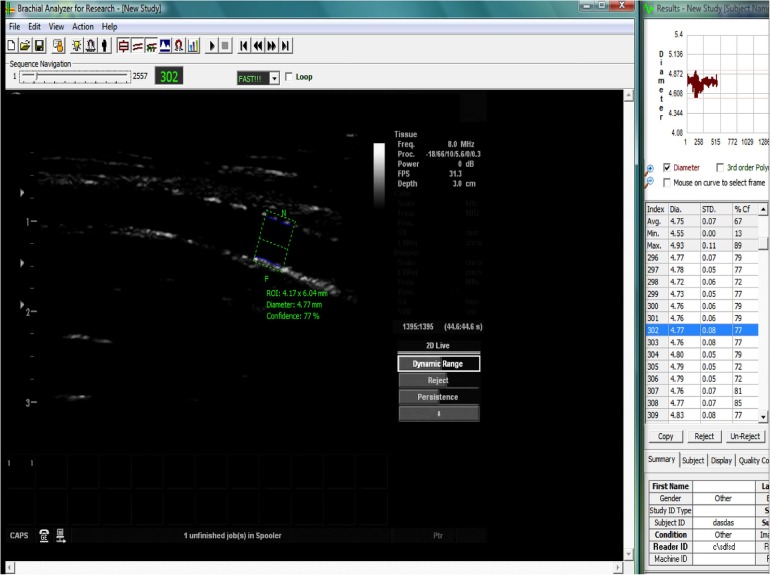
Screen shot of the MIA-IIc brachial analyser software recording the diameter of the brachial artery using B mode ultrasound, by identification of the lines of Pignoli in real time (green box=region of interest, blue lines=endothelium tracker).
